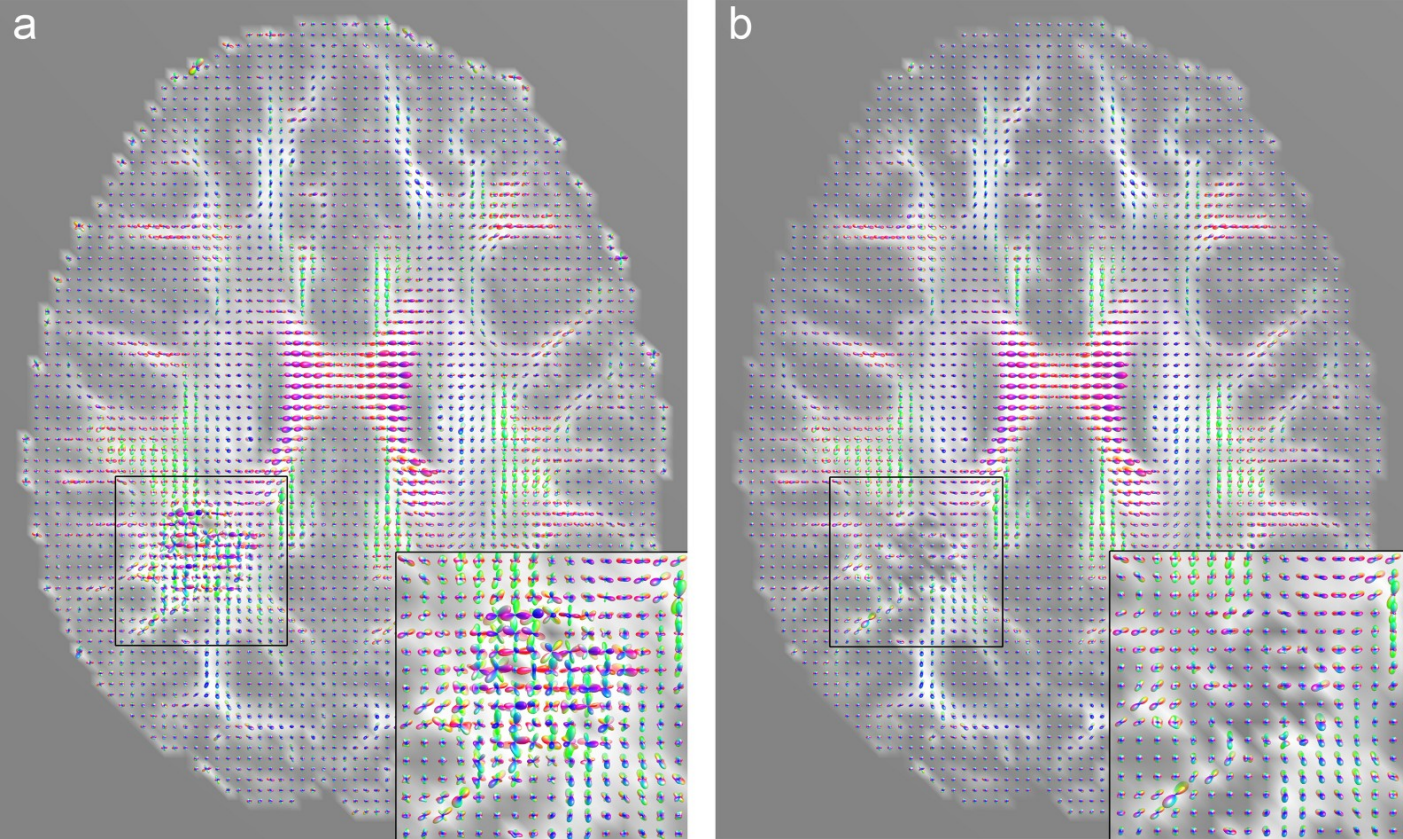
Effect of denoising DW-MRI data from patient B on CSA-ODFs. To provide anatomic context, GFA slice images are used as the background for the CSA-ODF glyphs. Images (a) and (b) both show the same slice, calculated with Matlab. The marked and enlarged areas show the tumor region inside the right hemisphere. (a) CSA-ODFs calculated from noisy DW-MRI data. (b) CSA-ODFs calculated after application of the OLPCA noise reduction scheme. Noise reduction was applied to the entire DW-MRI dataset but is most effective in regions with a low SNR, such as the marked and enlarged tumor region.
